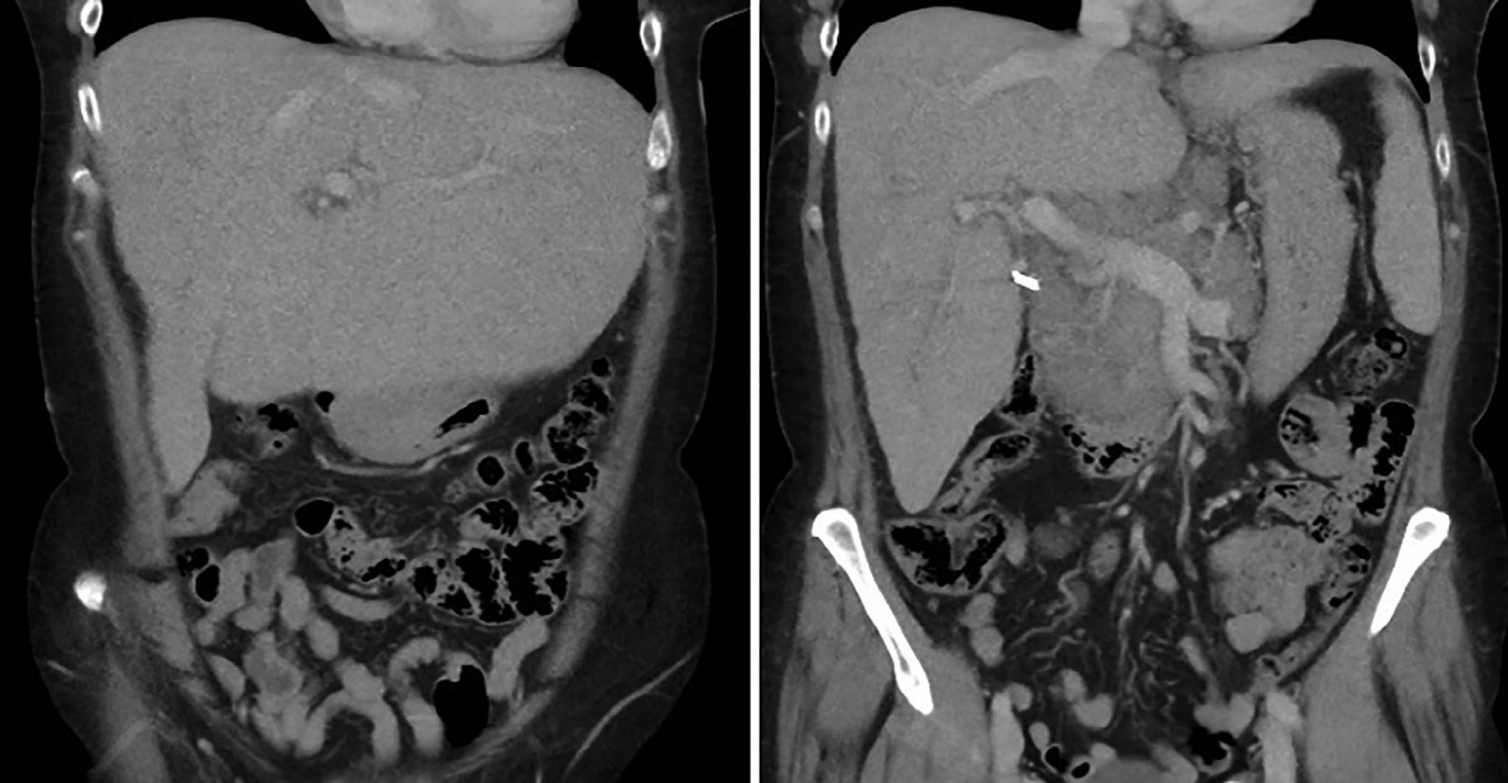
Computed tomography abdomen‐pelvis demonstrated hepatomegaly with a craniocaudal span of 23 cm, splenomegaly measuring 14 cm, and a soft tissue mass at the porta hepatis consistent with confluent lymphadenopathy with several other enlarged lymph nodes in the region of the coeliac axis and upper para‐aortic region measuring up to 35 mm.
